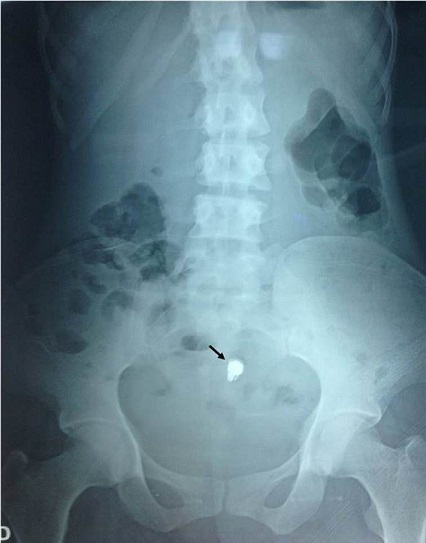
Plain abdominal radiography showing retained videocapsule (arrow)
